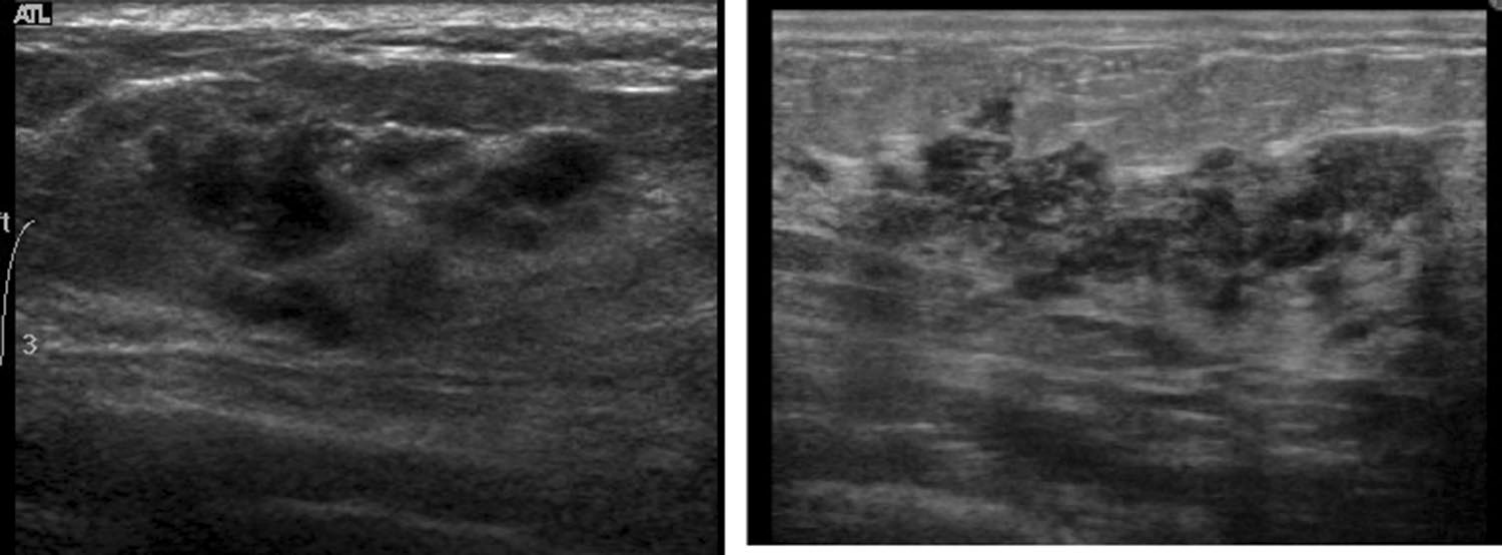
Two cases of ductal carcinoma in situ (DCIS). Ultrasound shows geographic hypoechoic areas in the mammary gland
